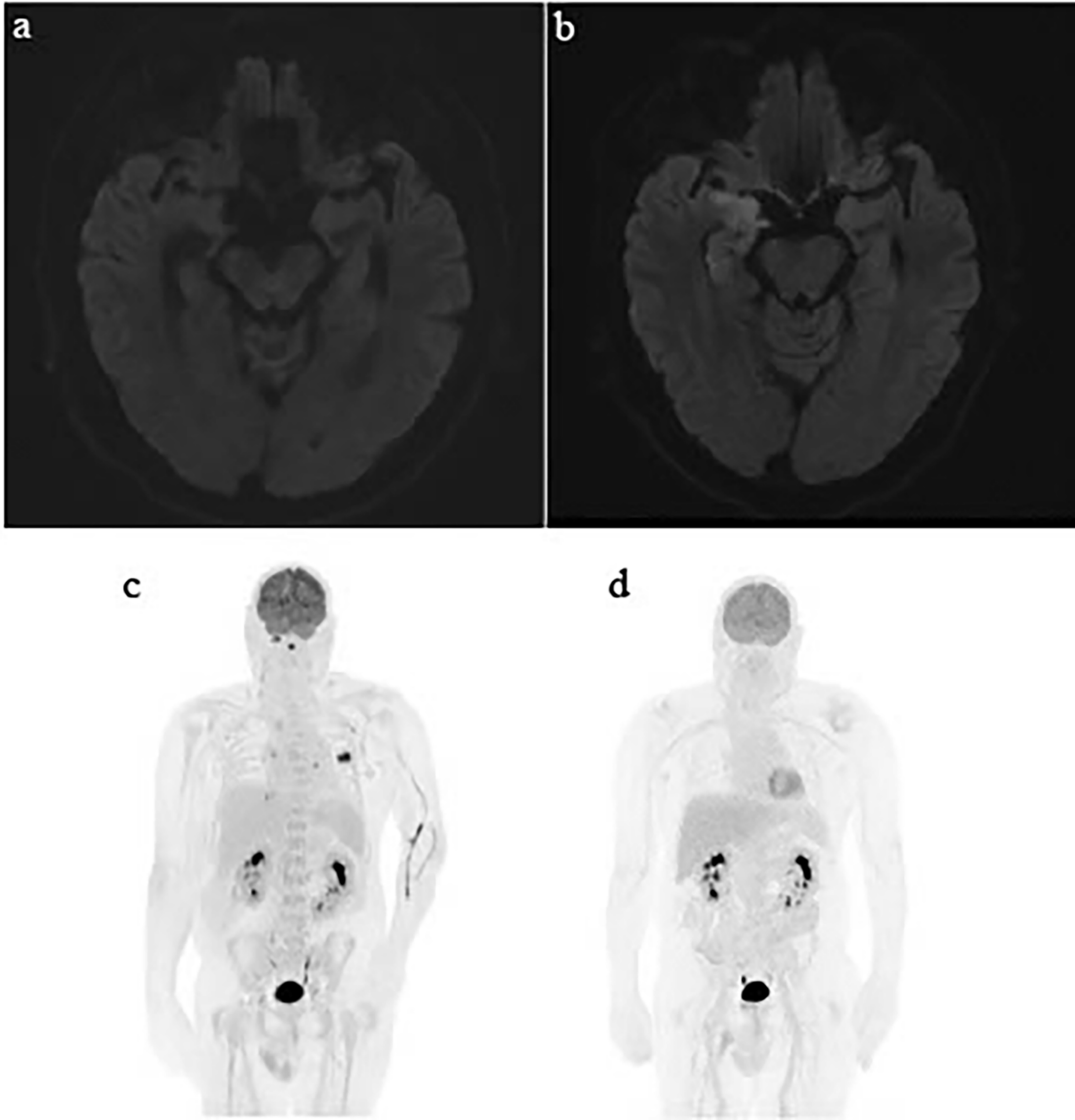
Serial MRI brain showing development of encephalitis and serial PET/CT demonstrating the patient’s durable response to immunotherapy. (A) MRI brain with gadolinium Sept 2, 2020—no abnormalities in medial temporal region. (B) MRI brain October 29, 2020 shows new T2/FLAIR hyperintensity in the right medial temporal lobes. (C) PET/CT March 2020. (D) PET CT March 2021.
